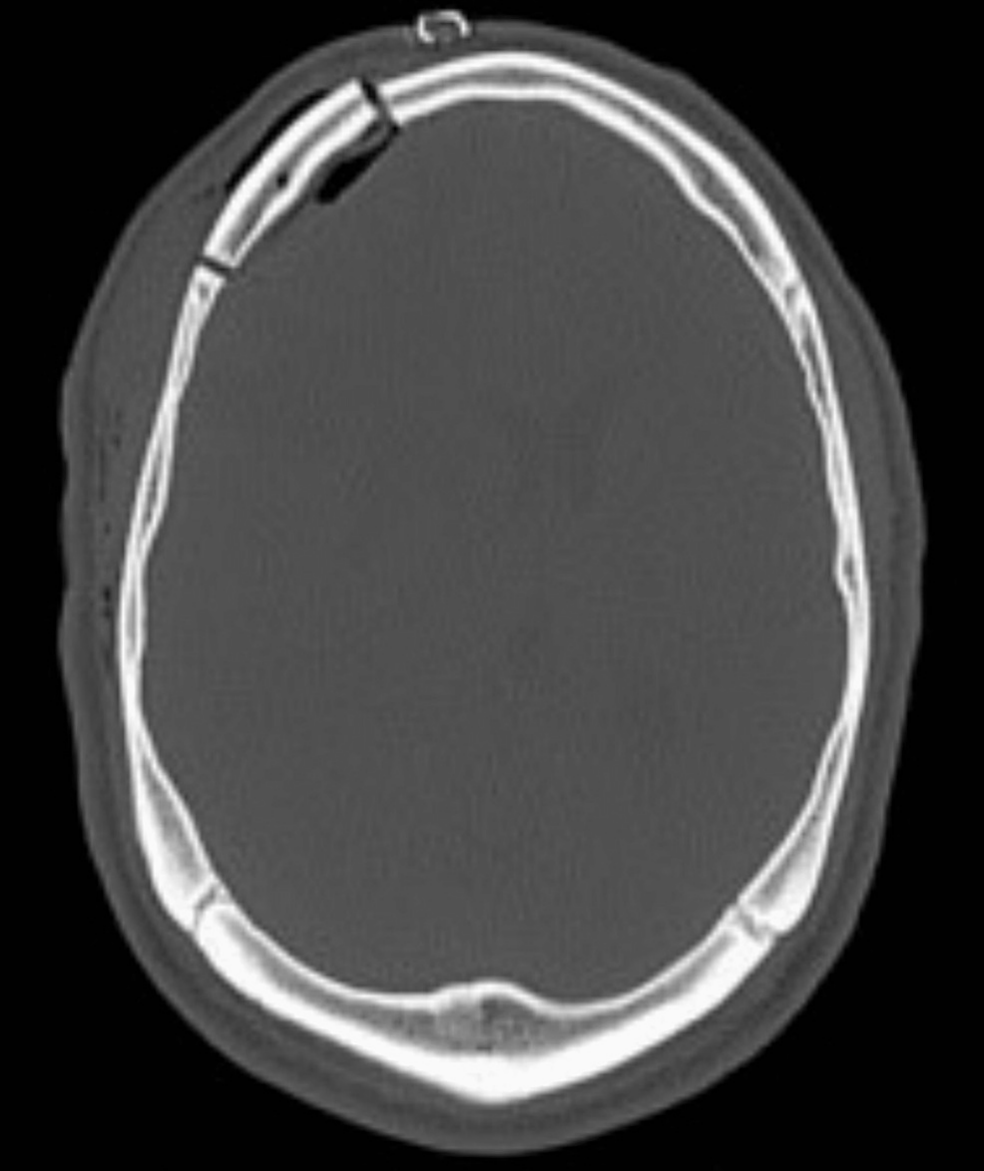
Axial CT demonstrating postsurgical changes after right frontal craniectomy.
